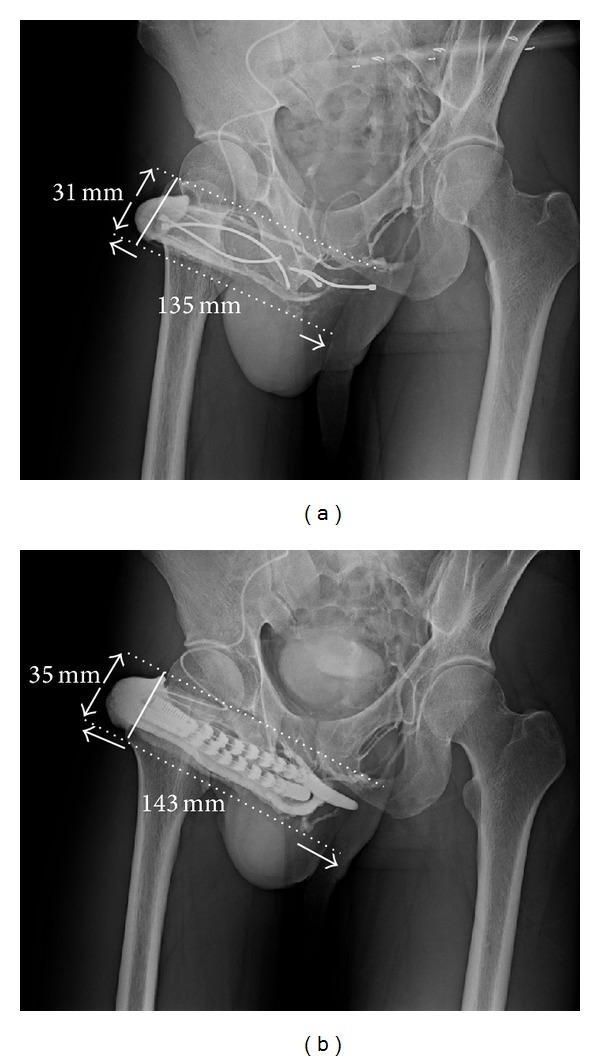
Pelvic X-ray film of 30° oblique view of a 35-year-old male of traumatic impotence. He underwent the first surgery somewhere in 2006. A mechanical failure of penile prosthesis prompted him to receive an implant revision and the venous ligation surgery for cold glans syndrome. (a) The glanular radius was enhanced from 31 mm to 35 mm after the penile venous surgery. (b) The DDV was ligated at the level of retrocoronal and hilum region. Enhancement was shown in both the glans penis and entire penile shaft after a contract medium was injected to the glans penis via a #23 scalp needle.
